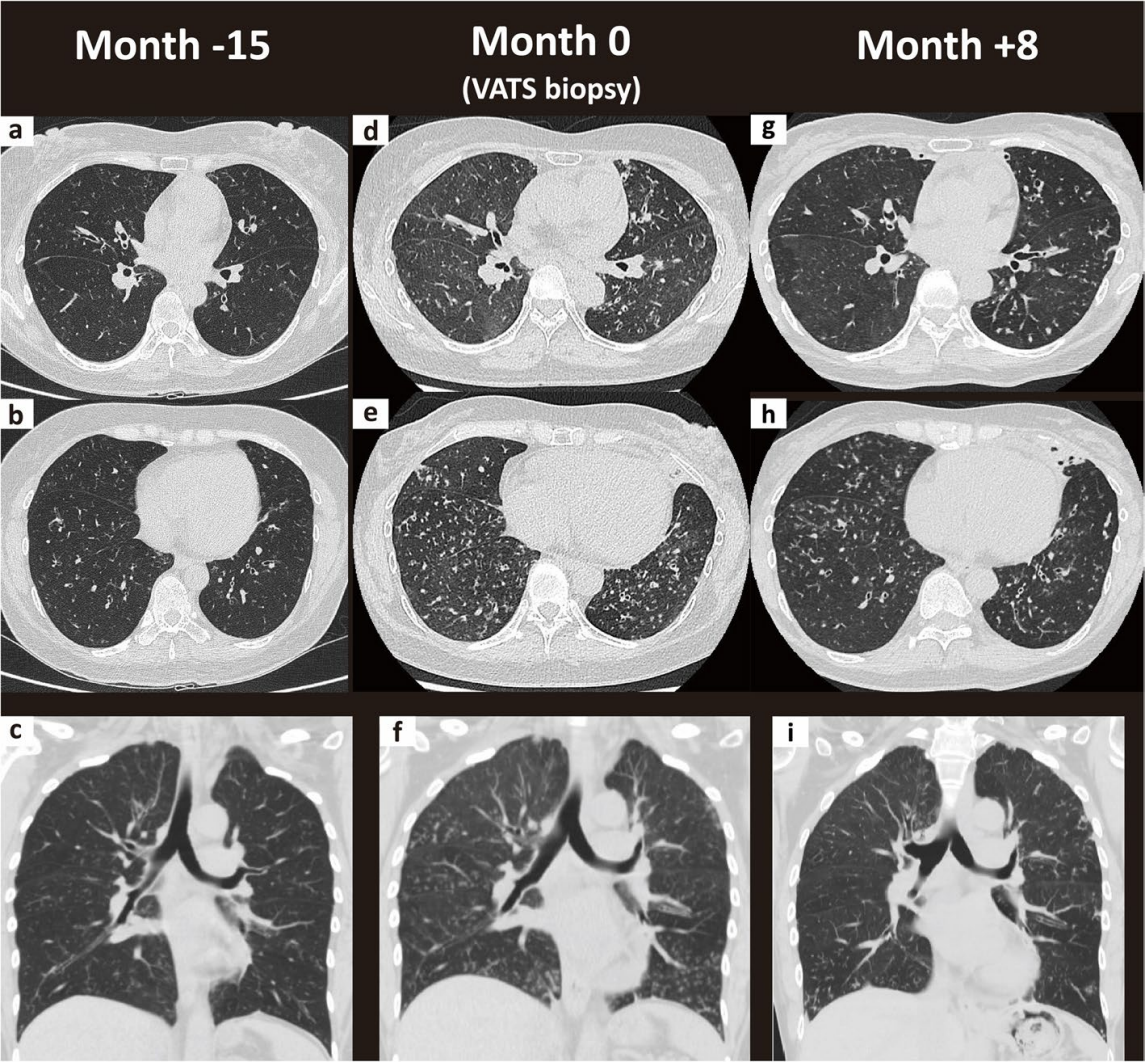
Axial and coronal views of chest computed tomography scan. a, b, c At 15 months before this presentation. d, e, f At the time of admission for video-assisted thoracoscopic surgery (VATS) biopsy. g, h, i At 8 months after DPB diagnosis, in non-exacerbation phase
